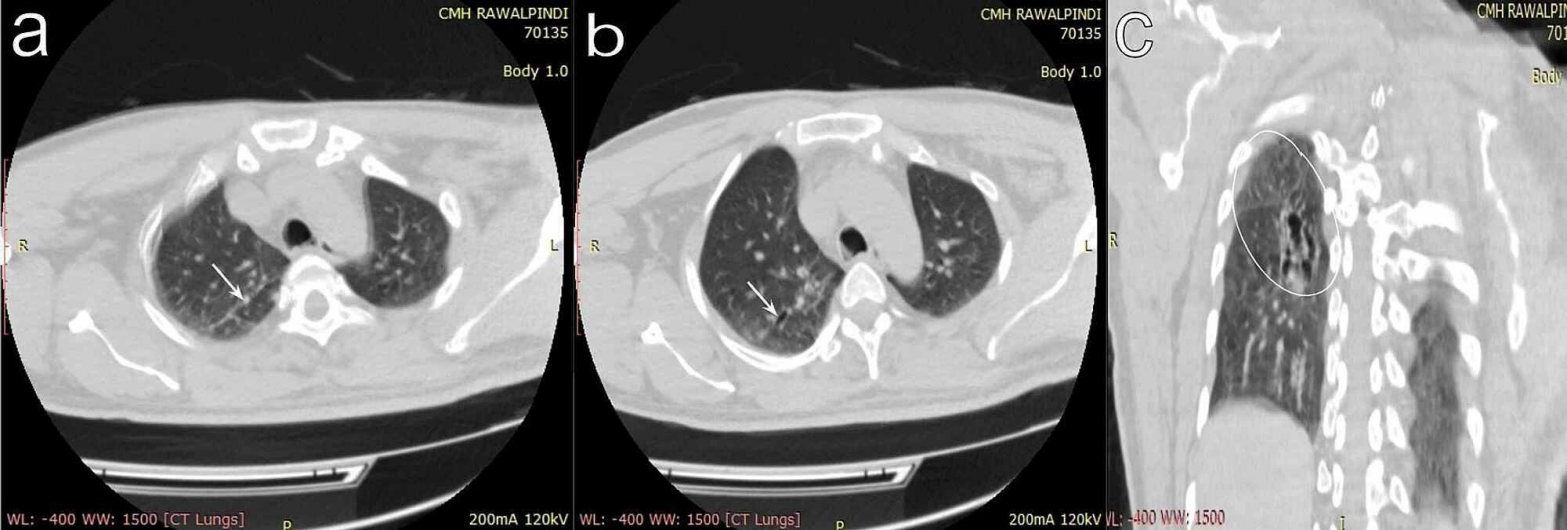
Thoracic HRCT shows a moderately bronchiectatic right lung attributable to old resolved tuberculosis a: a fibrotic band in the apical segment of the right upper lobe (arrow) on axial view.
